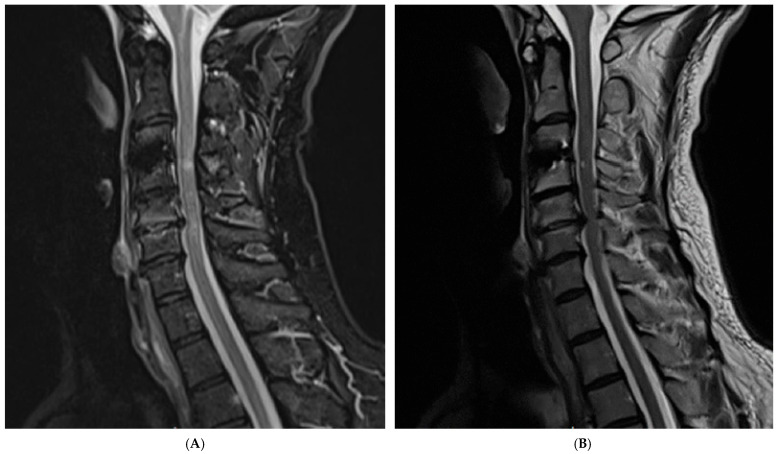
6-month postoperative MR images demonstrating resolution of infection and stable fusion. (A) Sagittal T2-weighted MRI showing complete resolution of prevertebral soft-tissue edema and absence of residual fluid collection, with normalization of surrounding anatomical structures. (B) Sagittal T1-weighted MRI confirming maintained position of the interbody implant without abnormal enhancement or evidence of recurrent abscess formation. These findings correlate with the patient’s clinical recovery, resolution of dysphagia, and return to neurological baseline.
